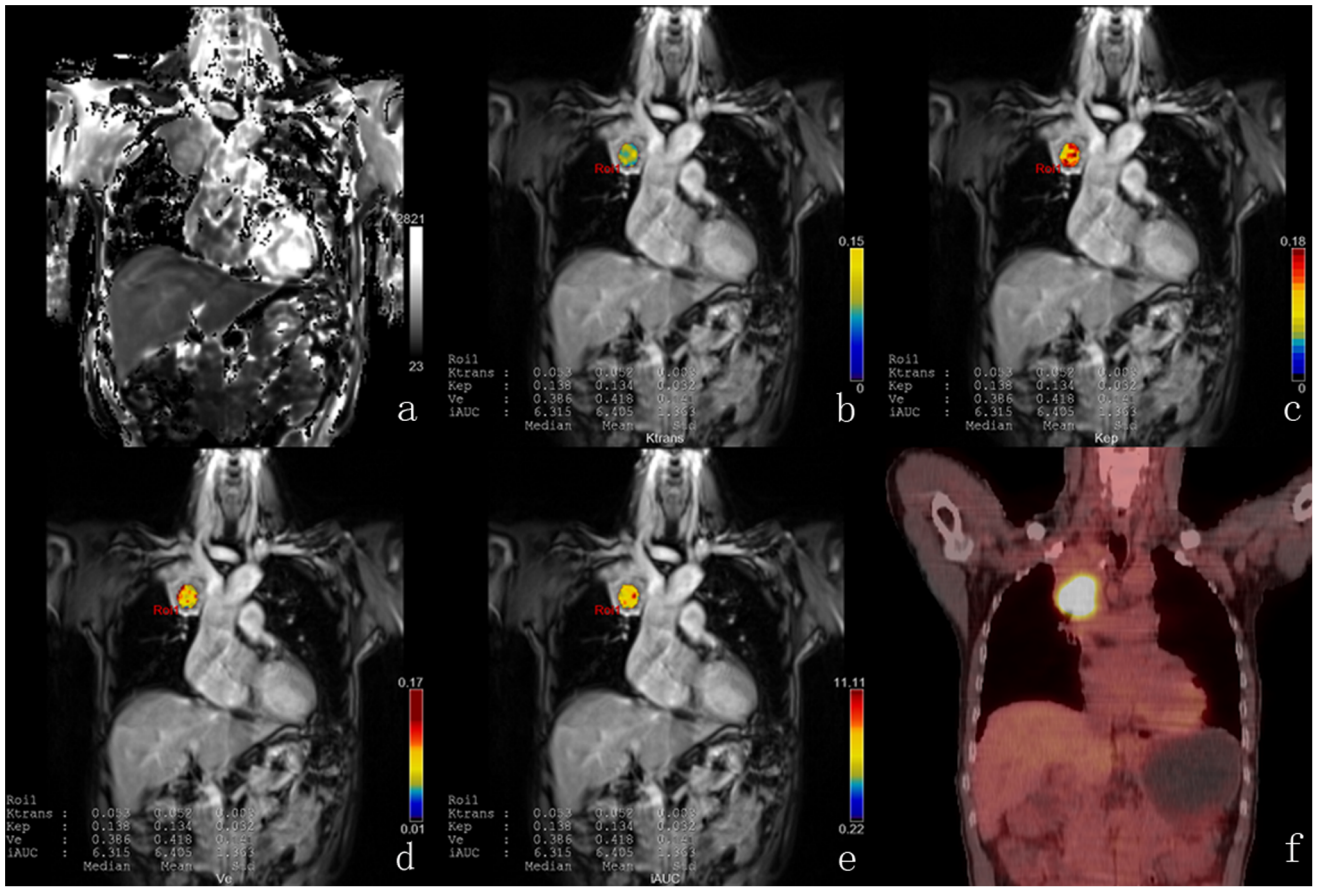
DCE-MRI and PET/CT of a representative case with SCC. T1 map (a) is automatically calculated by the precontrast images. Color parametric maps of Ktrans (b), Kep (c), Ve (d) and iAUC (e) based on coronal T1- weighted images of the chest demonstrate the increased tumor microcirculation parameters on the right upper lung. The corresponding PET/CT image (f) shows the avid glucose uptake in the tumor site.
